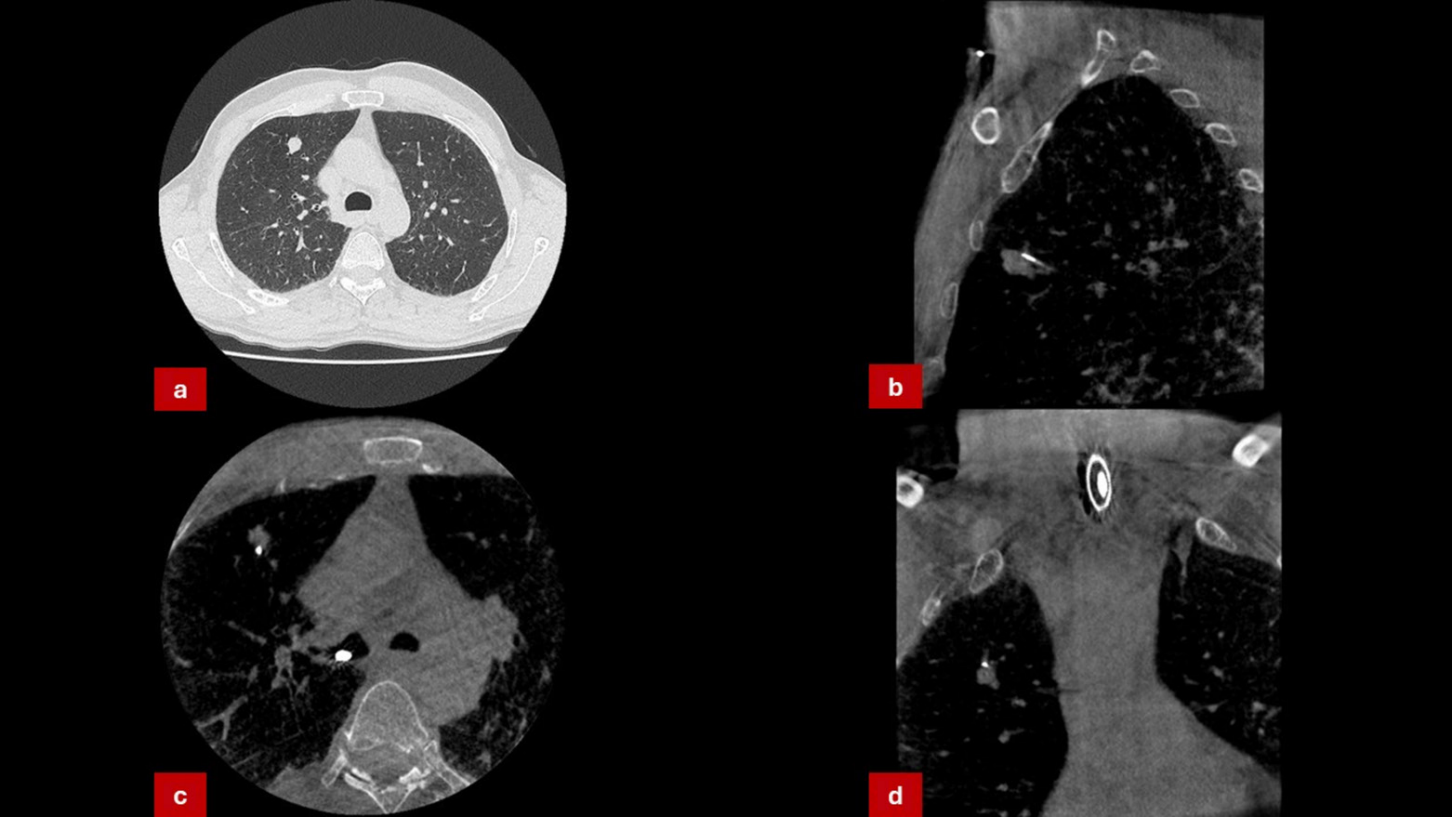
(a) CT‐scan showing solitary lesion in the right upper lobe. (b–d) CBCT‐Imaging confirming precise placement of biopsy tool (1.1 mm cryoprobe) within the targeted lesion.
